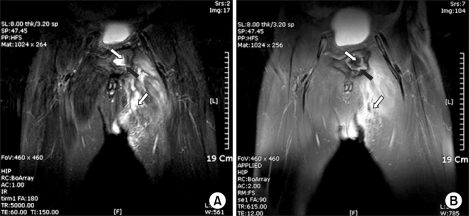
MRI shows an abscess (white arrow) within the deformed pubic ramus and the adductor longus, extending to the subcutaneous tissue, and osteomyelitis (black arrow) in the adjacent bone.
